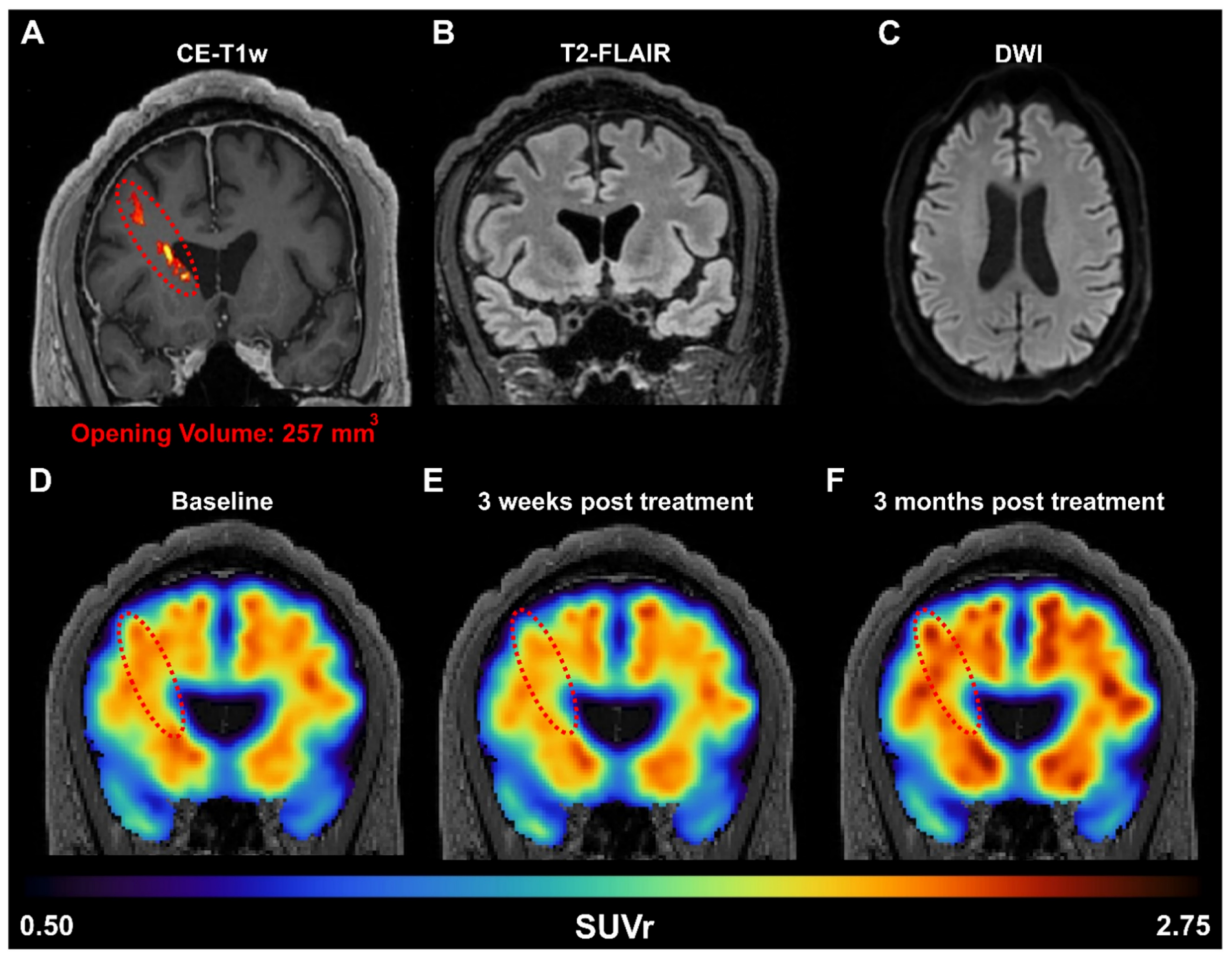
(A) Amyloid PET signal reduction following FUS treatment in an early AD patient. BBB opening in the right prefrontal cortex was confirmed immediately after FUS exposure through contrast enhanced T1-weighted (CE-T1w) MRI, revealing a contrast enhancement volume of 257 mm^3^ (red dotted oval). MRI safety scans, acquired on the day of treatment, including (B) T2-FLAIR and (C) DWI, indicate a safe opening without edema or hemorrhaging. The amyloid PET signal was measured at the (D) baseline pre-FUS, (E) 3 weeks, and (F) 3 months post-FUS. Within the treated frontal lobe, we detected a reduction of 1.8% in the SUVr 3 weeks after treatment, followed by a 5.9% increase in SUVr 3 months after treatment, all relative to the baseline scan.
